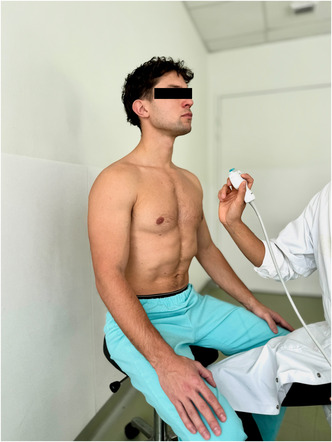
Initial position. For the initial shoulder ultrasound setup, the patient is seated while the examiner positions themselves very close, almost in a staggered alignment, placing 1 knee between the patient's 2 knees. The patient stays in a relaxed position with the back straight and both arms resting naturally. A linear ultrasound transducer is used for the examination. This setup provides a stable, comfortable position for both the patient and the examiner, facilitating accurate and reproducible evaluation of the supraspinatus tendon.
